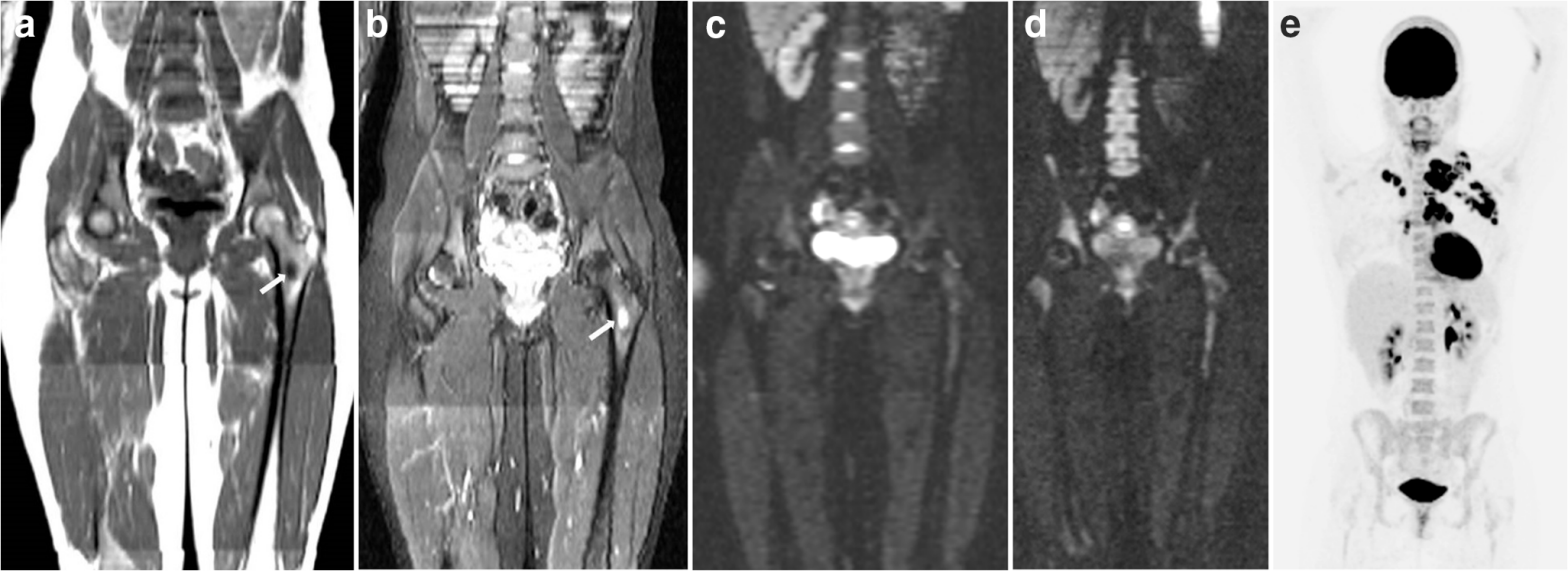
A 17-year-old girl with stage II Hodgkin lymphoma. Example in which the addition of DWI improved the agreement with the reference standard. Coronal T1-weighted MRI (a) shows a hypointense lesion in the left femur (arrow) that was interpreted as HL bone marrow involvement by the WB-MRI readers. At coronal T2-weighted MRI (b), the lesion appears as a hyperintensity (arrow). Coronal DWI (b100 (c) and 800 (d) shows no restricted diffusion in the left femur, and thus, no bone marrow involvement was reported by the readers for the WB-MRI reading including DWI. Coronal maximum intensity projection (MIP) of the FDG-PET (e) shows involvement of the cervical, axillary, infraclavicular and mediastinal lymph node stations, but no signs of bone marrow involvement are present
